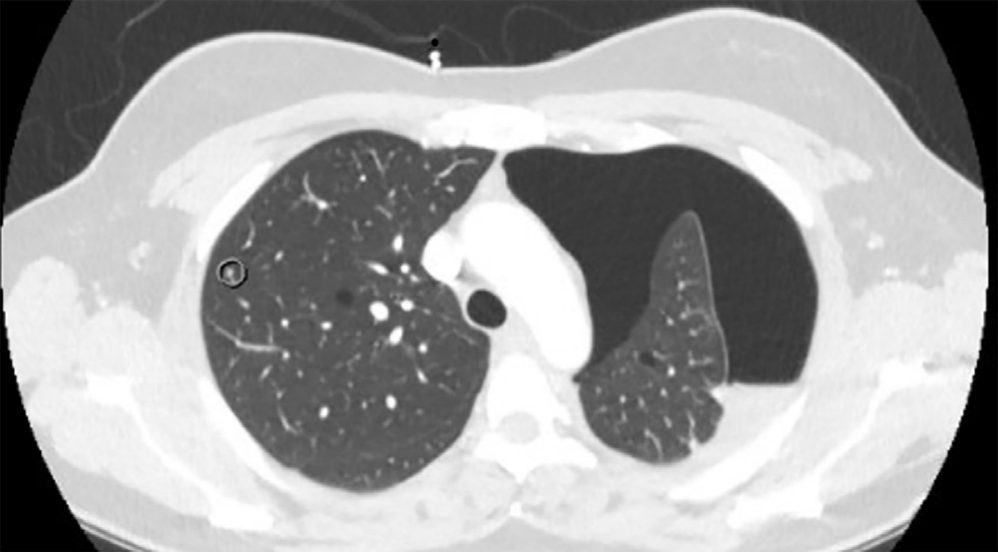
Chest CT showing left hydropneumothorax with a large gas component and multiple thin-walled bilateral pulmonary parenchymal cysts. The cystic changes are characteristic of pulmonary lymphangioleiomyomatosis (LAM). The circled region highlights an area of significant parenchymal involvement, aiding in the diagnostic assessment.
